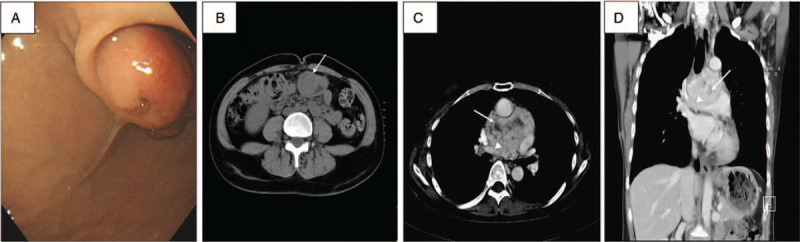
A–D, Esophagogastroduodenoscopy and computed tomography (CT) scan of the chest. A, Esophagogastroduodenoscopy showed a submucosal mass measuring 4 × 2.5 × 2.5 cm in size, with focally ulcerated mucosa. B, A CT scan of the abdomen depicted a 5 × 3.5 cm well-defined hypervascular tumor at the lower gastric body. C, D, Axial and sagittal images of the chest depict a 7.4 × 6.6 cm hypervascular and heterogeneous mass in the precarinal region (arrows) with the encasement of Right pulmonary artery (arrowheads).
